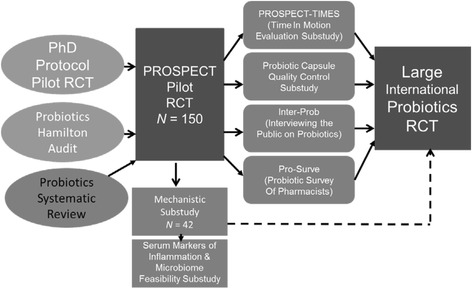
The probiotics in ICU research program. The PROSPECT pilot trial is part of the probiotics in ICU Research Program
